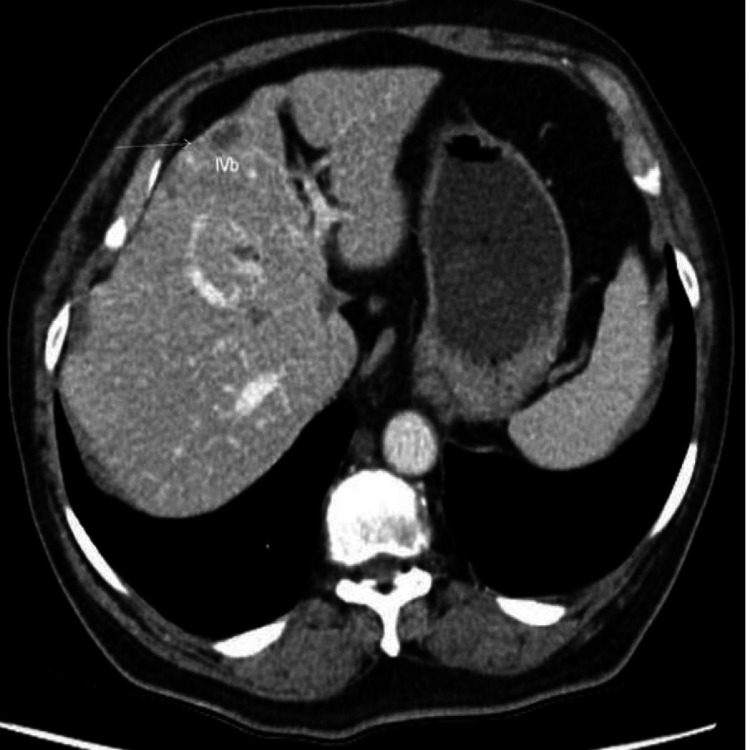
CT abdomen cross-sectional view triple-phase (triphase) The image shows complete resolution of stomach cancer with complete disappearance of metastasis along the liver and portal area six years after the initial presentation (white arrow) CT: computed tomography; IV b: segment IV (inferior) lateral to the falciform ligament
